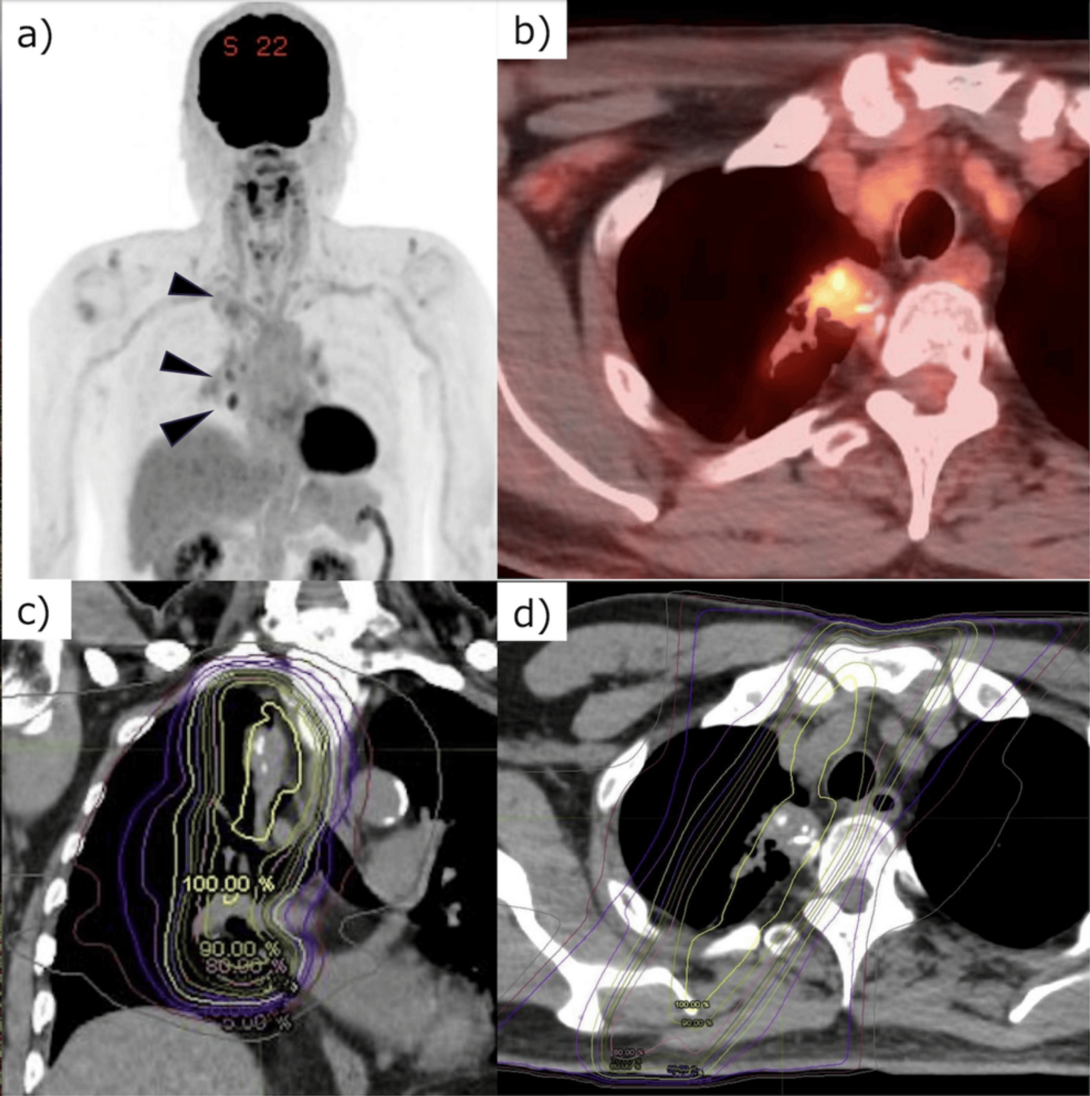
A treatment plan for clinical stage T2aN2M0 right upper lobe lung cancer using three-dimensional conformal radiotherapy 18-Fluorine fludeoxyglucose positron emission tomography/computed tomography (PET/CT) was performed for lung cancer staging in an 83-year-old male. (a) Maximum intensity projection image showing accumulation in the right upper lobe and ipsilateral mediastinal lymph nodes (black arrow heads). (b) Axial image of the primary tumor on PET/CT, and the biopsy confirmed an adenocarcinoma. A treatment plan was developed for the clinical stage T2aN2M0 right upper lobe lung cancer using three-dimensional conformal radiotherapy. The total dose was 66 Gy in 33 fractions, and the treatment plan was modified at 40 Gy to reduce the dose to the spine and lungs. Panels (c) and (d) show coronal and axial sections of the combined first and second plan dose distribution maps.
